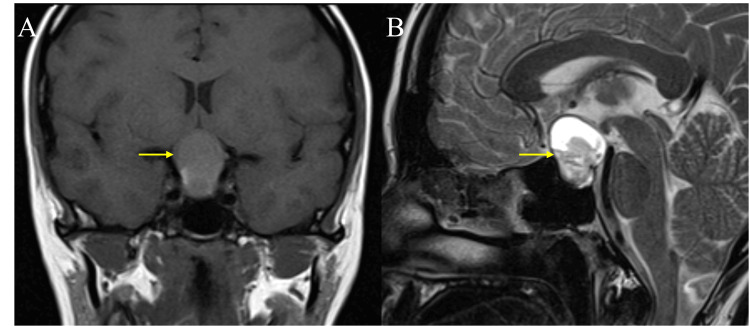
The MRI of the sella turcica shows a well-demarcated solid and cystic sellar mass with suprasellar extension and mass effect on the chiasm and third ventricle. The solid component has a heterogenous appearance on T2 (A) (yellow arrow) and a slightly hyperintense signal on T1 (B) (yellow arrow). All the features indicate a diagnosis of a xanthogranuloma.
